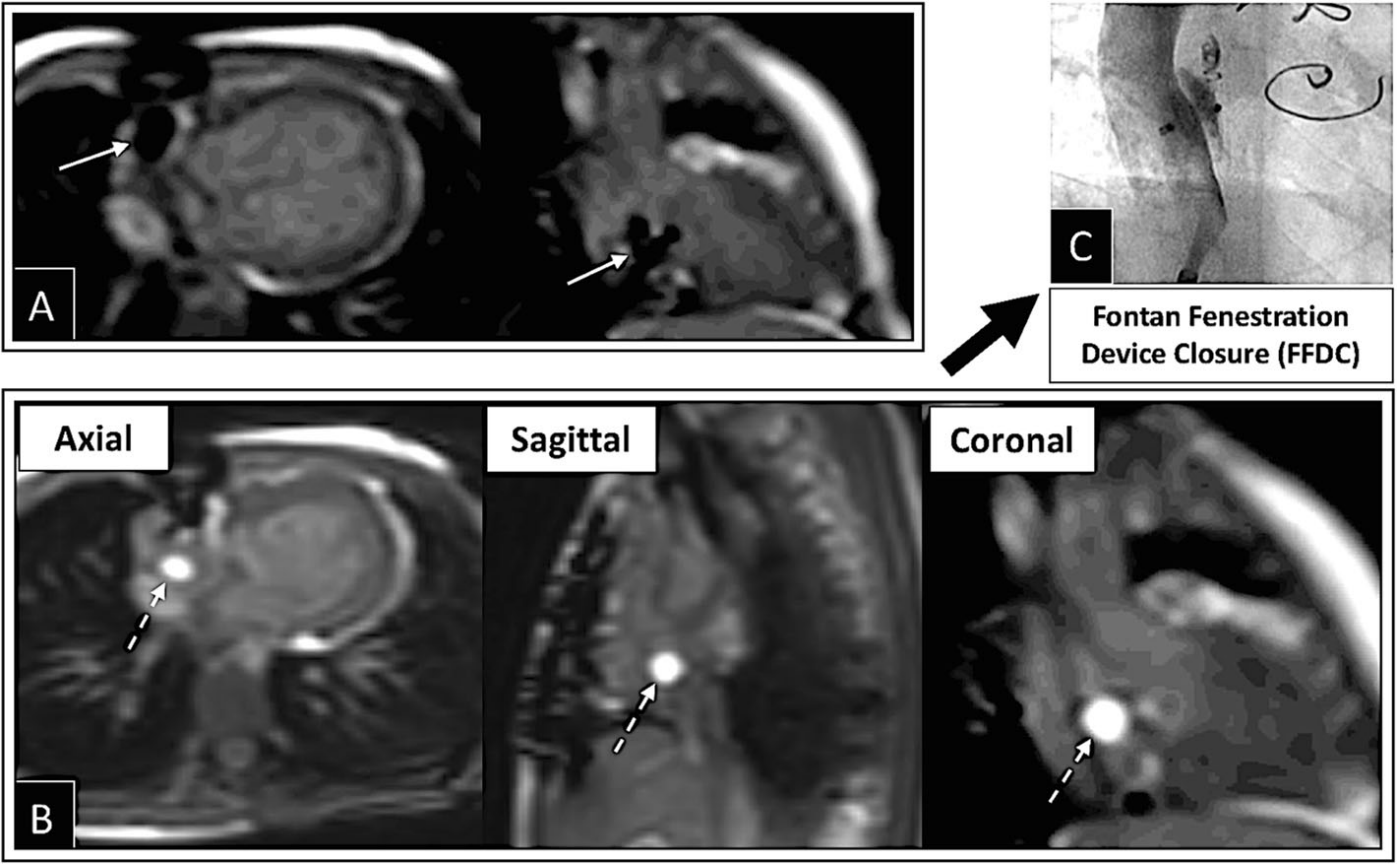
Depicts successful iCMR Fontan fenestration test occlusion (FFTO) followed by successful Fontan fenestration device closure (FFDC) in the x-ray fluoroscopy cath lab. a Under iCMR, the Fontan fenestration (FF) was crossed using an MR-conditional guidewire. An MR-conditional guidewire is seen across the Fontan fenestration and in the RA appendage. b The gadolinium-filled balloon was deflated to advance across the FF, inflated again in the right/pulmonary venous atrium and then pulled back to perform FFTO. Cath and CMR hemodynamics were repeated with FFTO. c If deemed appropriate, the subject was transferred to the x-ray fluoroscopy lab for FFDC. *Solid White Arrow– MR-conditional guidewire;Dashed White Arrow– Gadolinium-filled balloon*
